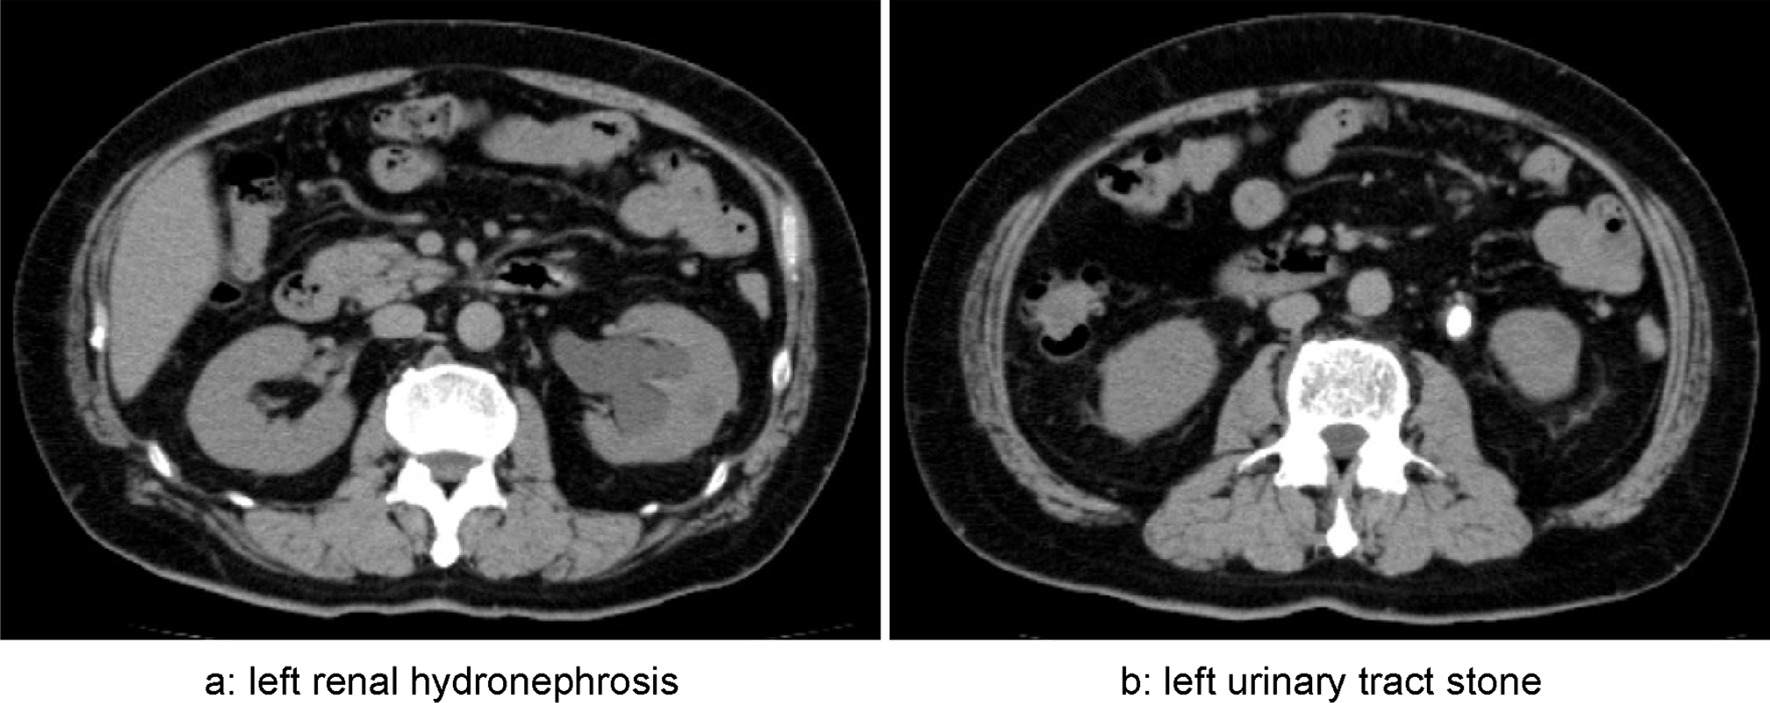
Plain thoracic, abdominal, and pelvic computed tomography. a left renal hydronephrosis. b left urinary tract stone
